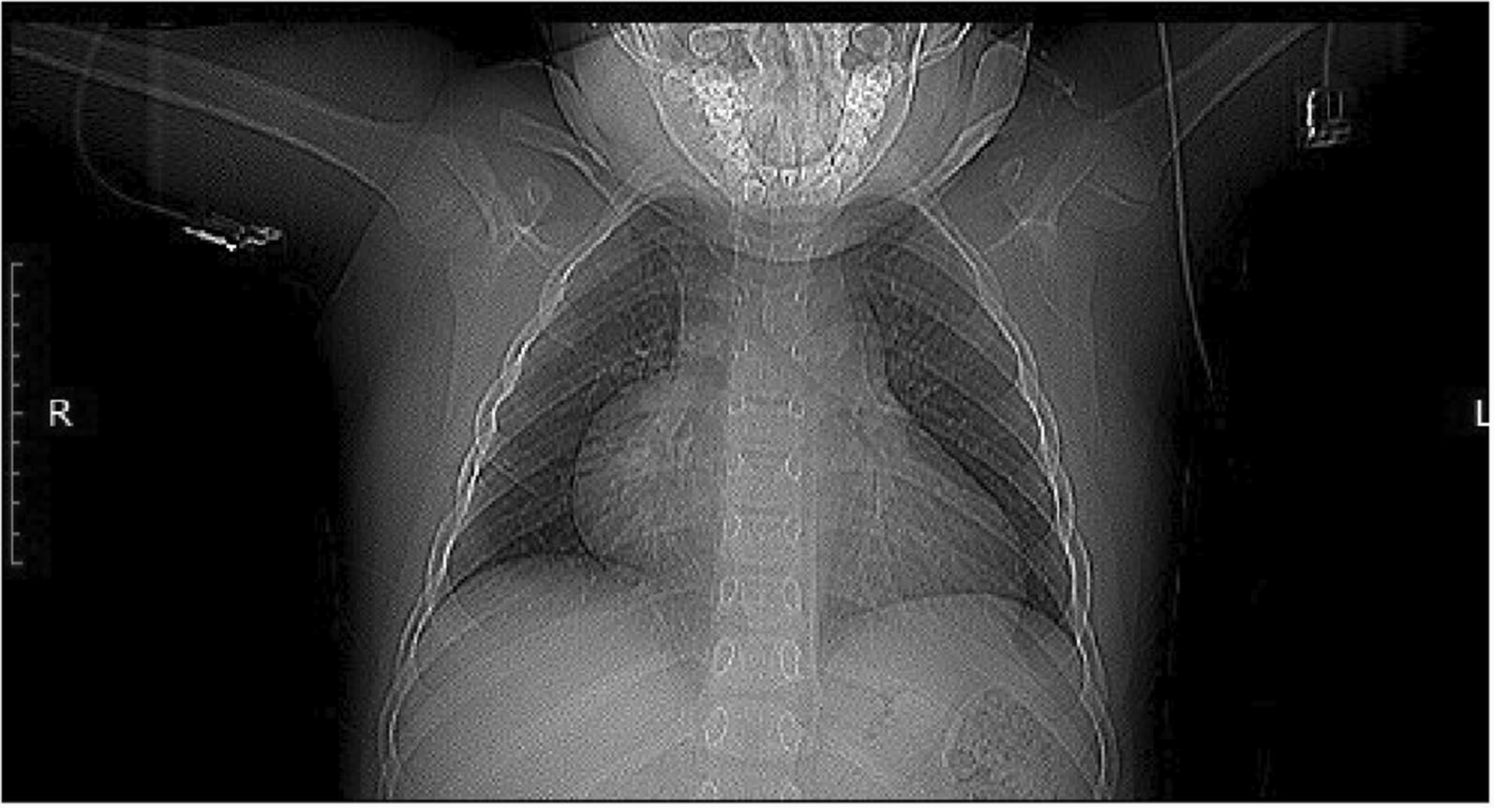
Pre-operative chest X-ray
